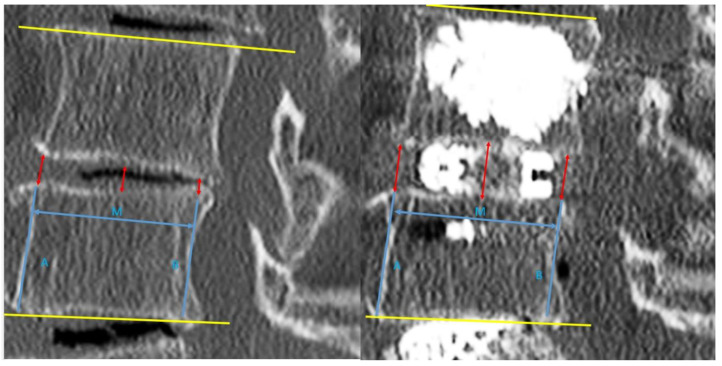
Mid sagittal cut of CT lumbar spine for measurement of parameters with left picture showed pre-operative cut and right picture showed post-operative cut of mid sagittal CT lumbar spine. Anterior vertebral line was drawn for caudal vertebra (line A) and anterior disc height was measured from the line parallel to line A from the caudal end plate to the cephalad end plate. The posterior vertebra line was drawn for caudal vertebra (line B) and posterior disc height is measured from line parallel to line A from caudal end plate to cephalad end plate. Middle disc height is measured at the end plate in the midpoint of lines A and B (M point) to the corresponding cephalad vertebral body end plate.
